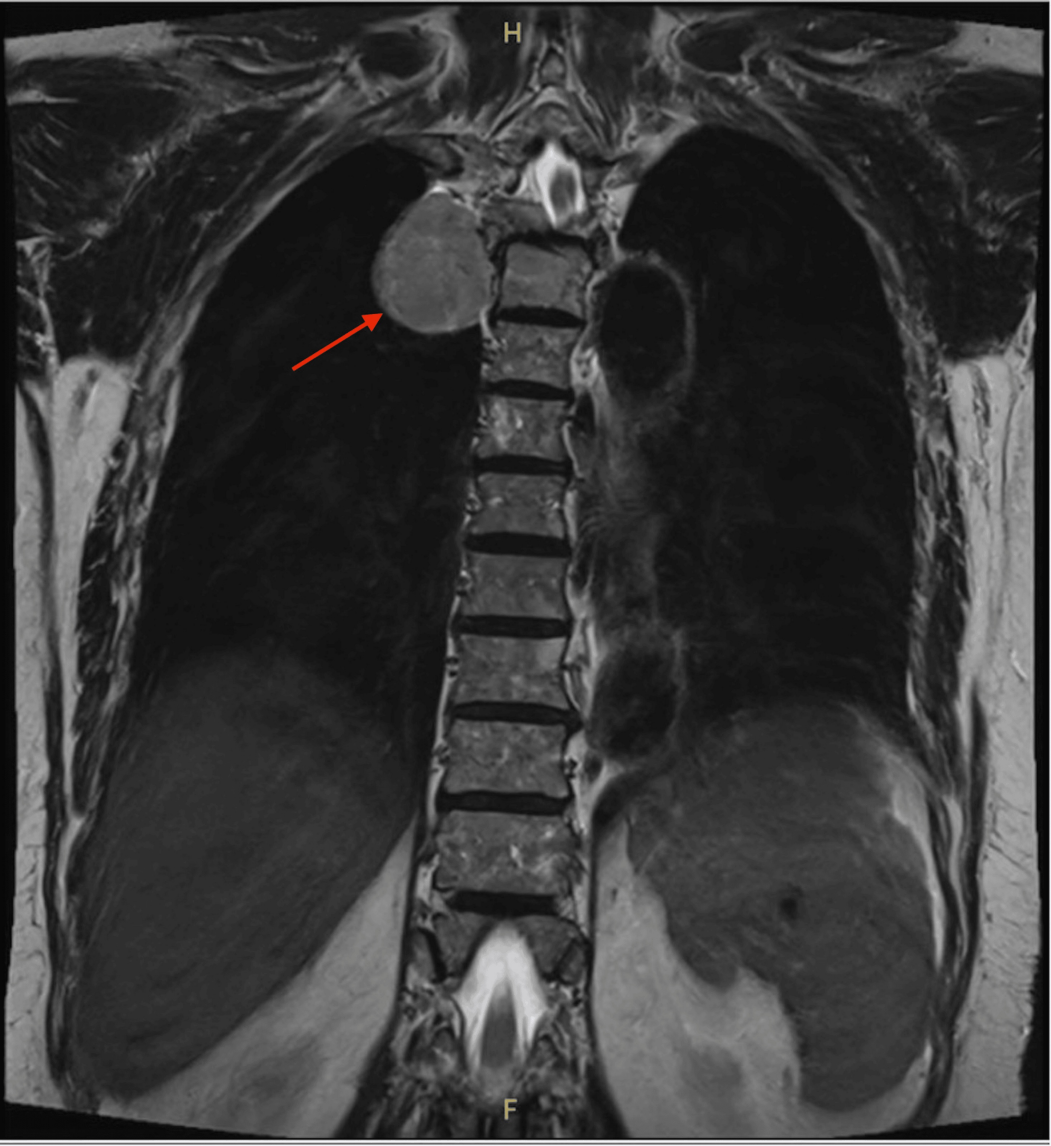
Coronal view of the thoracic MRI The red arrow shows a well-defined lobulated right-sided paraspinal mass at the thoracic vertebrae 3 to 5 (T3-T5) level, measuring 3.8 x 5.6 x 4.0 cm, with extension into the right side of the posterior mediastinum region in the thoracic cavity.
